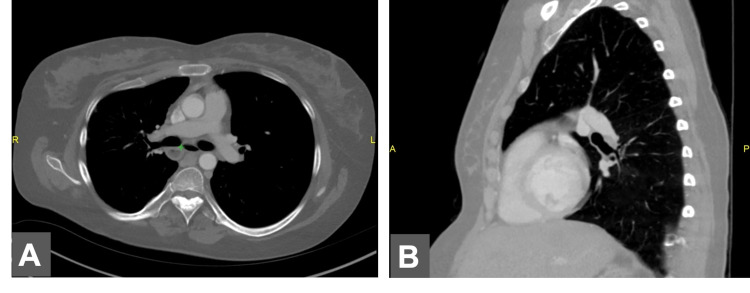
Chest CT images A. Axial chest CT image showing bronchi inferior to the pulmonary artery. B. Lateral chest CT image demonstrating hyperatrial left bronchus CT: computed tomography
